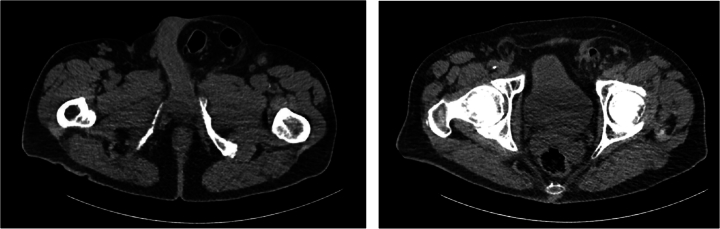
Left sided inguinal hernia on CT-abdomen eight months prior to presentation on the outpatient surgery clinic.
